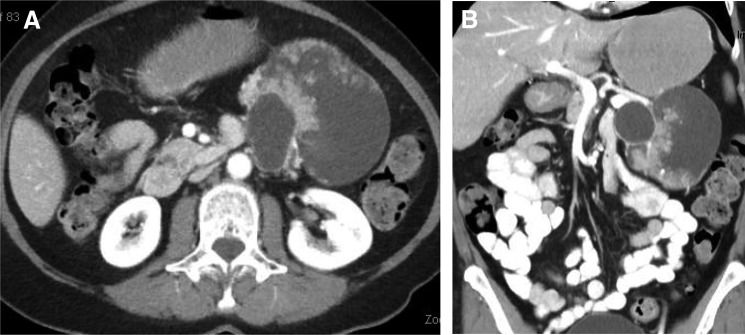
Radiographic appearance of cystic neoplasm in the tail of the pancreas. (A) Transverse and (B) coronal image of cystic neoplasm arising from the tail of the pancreas with solid and cystic components.
